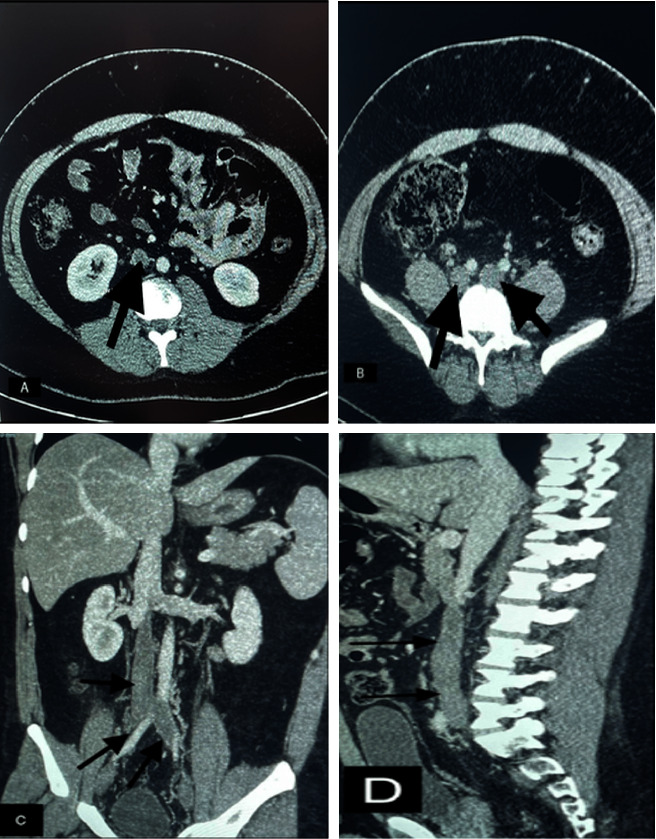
CT abdomen and pelvis with contrast. Large thrombus in the distal vena cava extending to the renal artery and both external and internal iliac veins.
